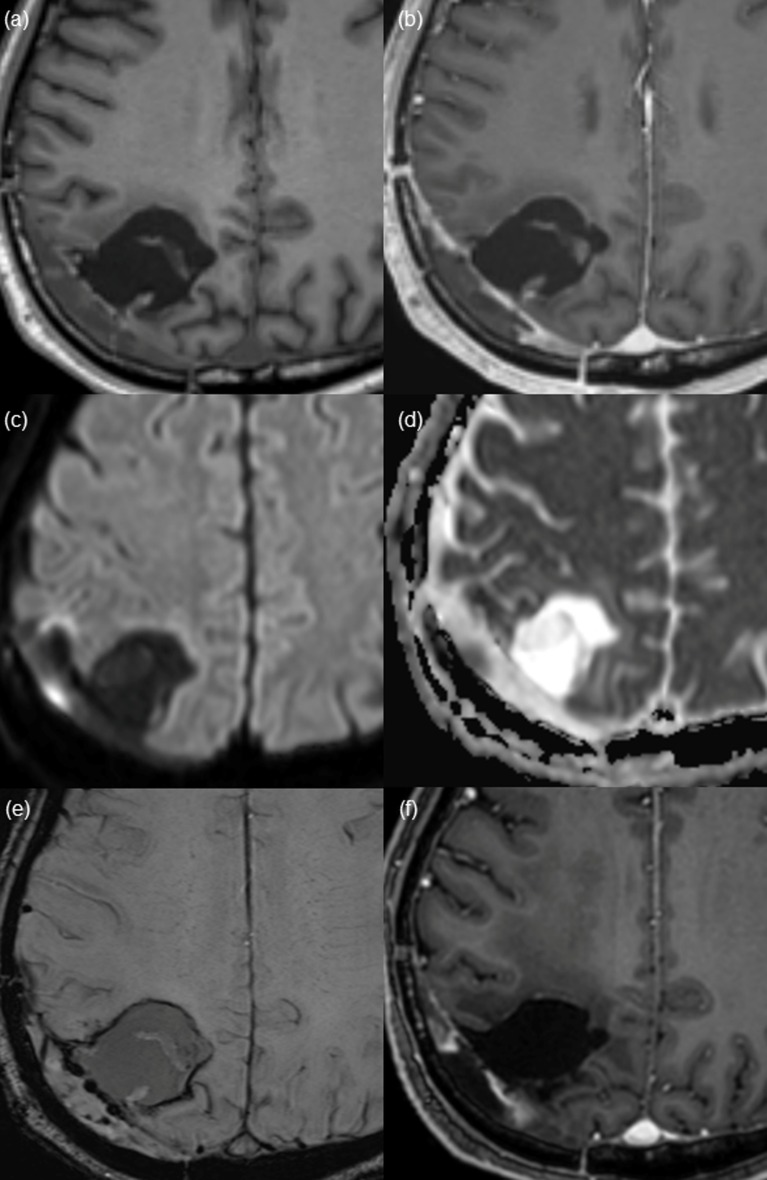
Thin wall enhancement pattern of the surgical cavity found on post-CCRT magnetic resonance (MR) imaging from a 71-year-old patient after gross total resection of glioblastoma. (a and b) Pre- and post-contrast enhanced T1 WI shows thin linear enhancement along the surgical cavity wall. (c) DWI and corresponding (d) ADC map suggest the possibility of diffusion restriction. However, considering the dark signal intensity on SWI (e), high SI on DWI reflects hemorrhage and does not suggest true diffusion restriction. (f) After 20 months, on the last follow up image, no measurable enhanced lesion is shown on contrast-enhanced T1WI.
